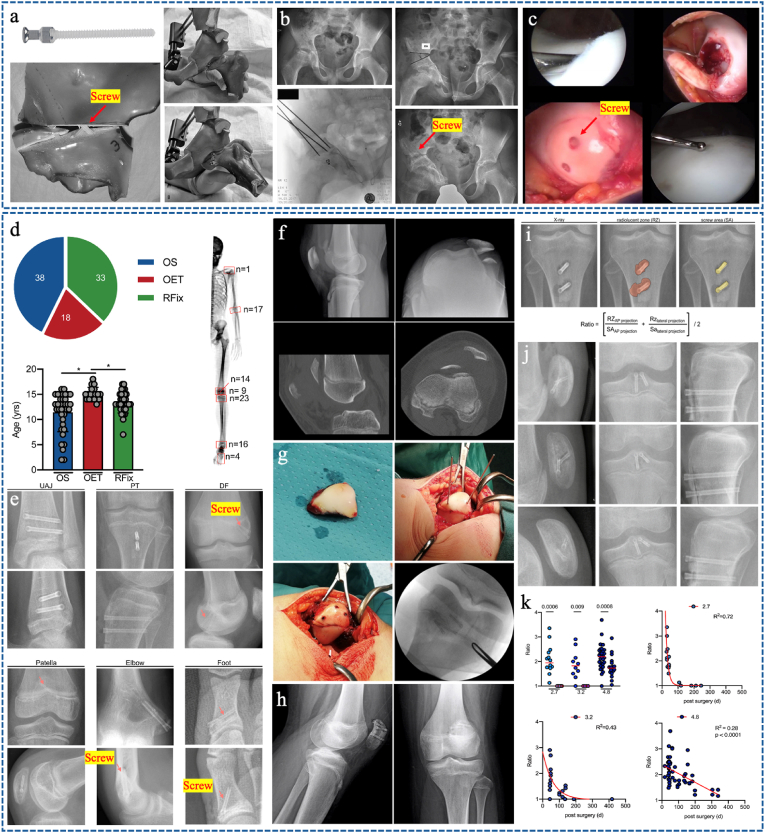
(a) Test stability of triple innominate osteotomy internally fixed with 2–3 bioabsorbable screws or steel screws. Reproduced with permission from Ref. [175]. Copyright 2007, Wilkins. (b) A 10.5-year-old boy with Legg-Calvé-Perthes disease conduct a triple osteotomy fixed with resorbable PLGA screws. Reproduced with permission from Ref. [160]. Copyright 2021, Wolters Kluwer Health. (c) A17-year-old boy diagnosed with osteochondritis dissecans of the lateral aspect of the trochlea and underwent open reduction and fixation with 2 bioabsorbable screws, second-look arthroscopic demonstrated fully healed. Reproduced with permission from Ref. [179]. Copyright 2019, SAGE. (d–e) Overview of the presented surgeries and the included patient cohort and implants at the different anatomical localizations. Reproduced with permission from Ref. [173]. Copyright 2021, Elsevier. (f–h) X-ray and CT showing a free osteochondral fragment along with lateral dislocation of the patella. Reproduced with permission from Ref. [180]. Copyright 2021, SAGE. (i–k) Evaluation of the radiolucent zone (RZ)/screw area (SA) ratio by use of standard radiographs to assess gas release from MAGNEZIX® CS 2.7 mm, CS 3.2 mm and CSC 4.8 mm Reproduced with permission from Ref. [182]. Copyright 2022, Springer.
